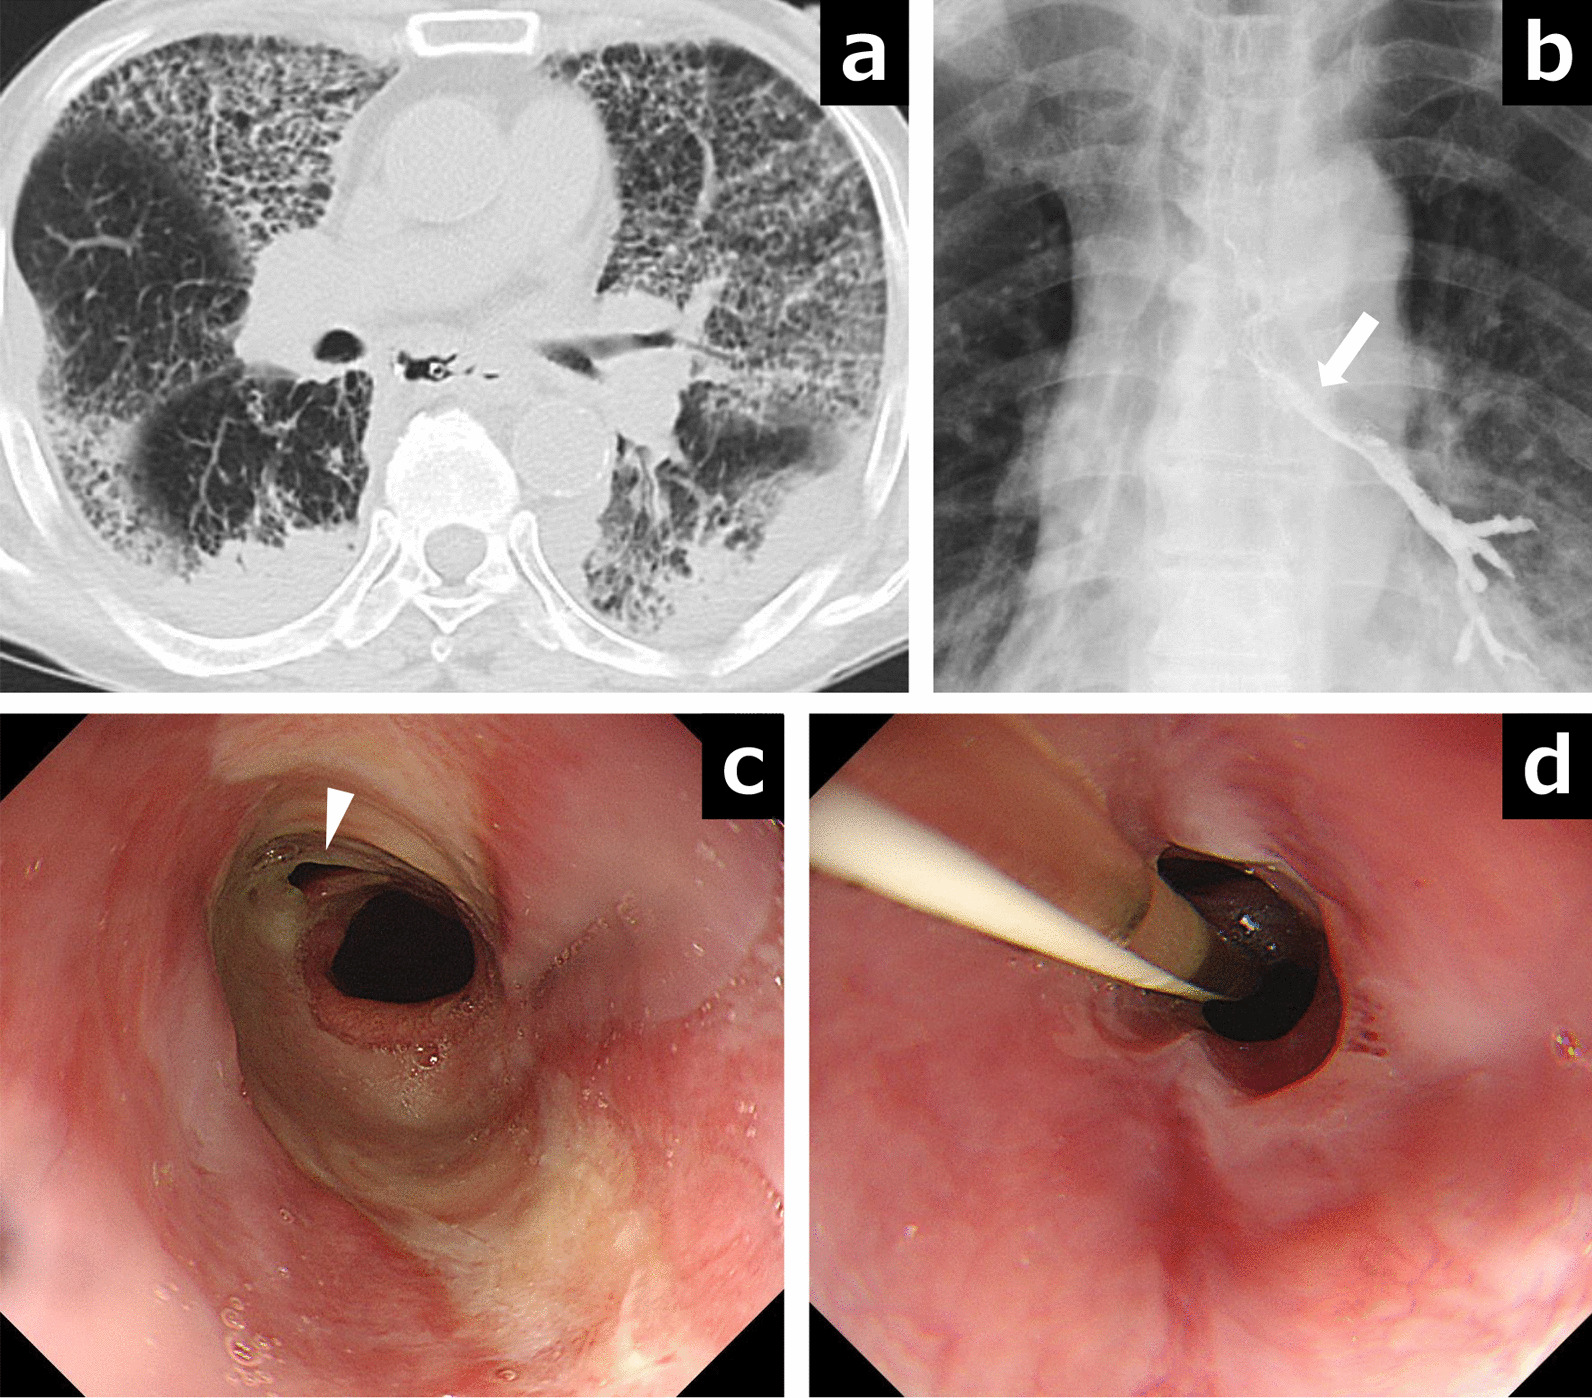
The findings of esophago-left bronchial fistula are shown. a CT images showed bilateral severe pneumonia. b Gastrografin contrast study showed leakage into the left main bronchus (white arrow). c A fistula (white arrowhead) was detected in the anterior wall of the esophagogastric anastomotic area by upper endoscopy. d The esophageal ulcer shrank, but the fistula did not close after 1 month
